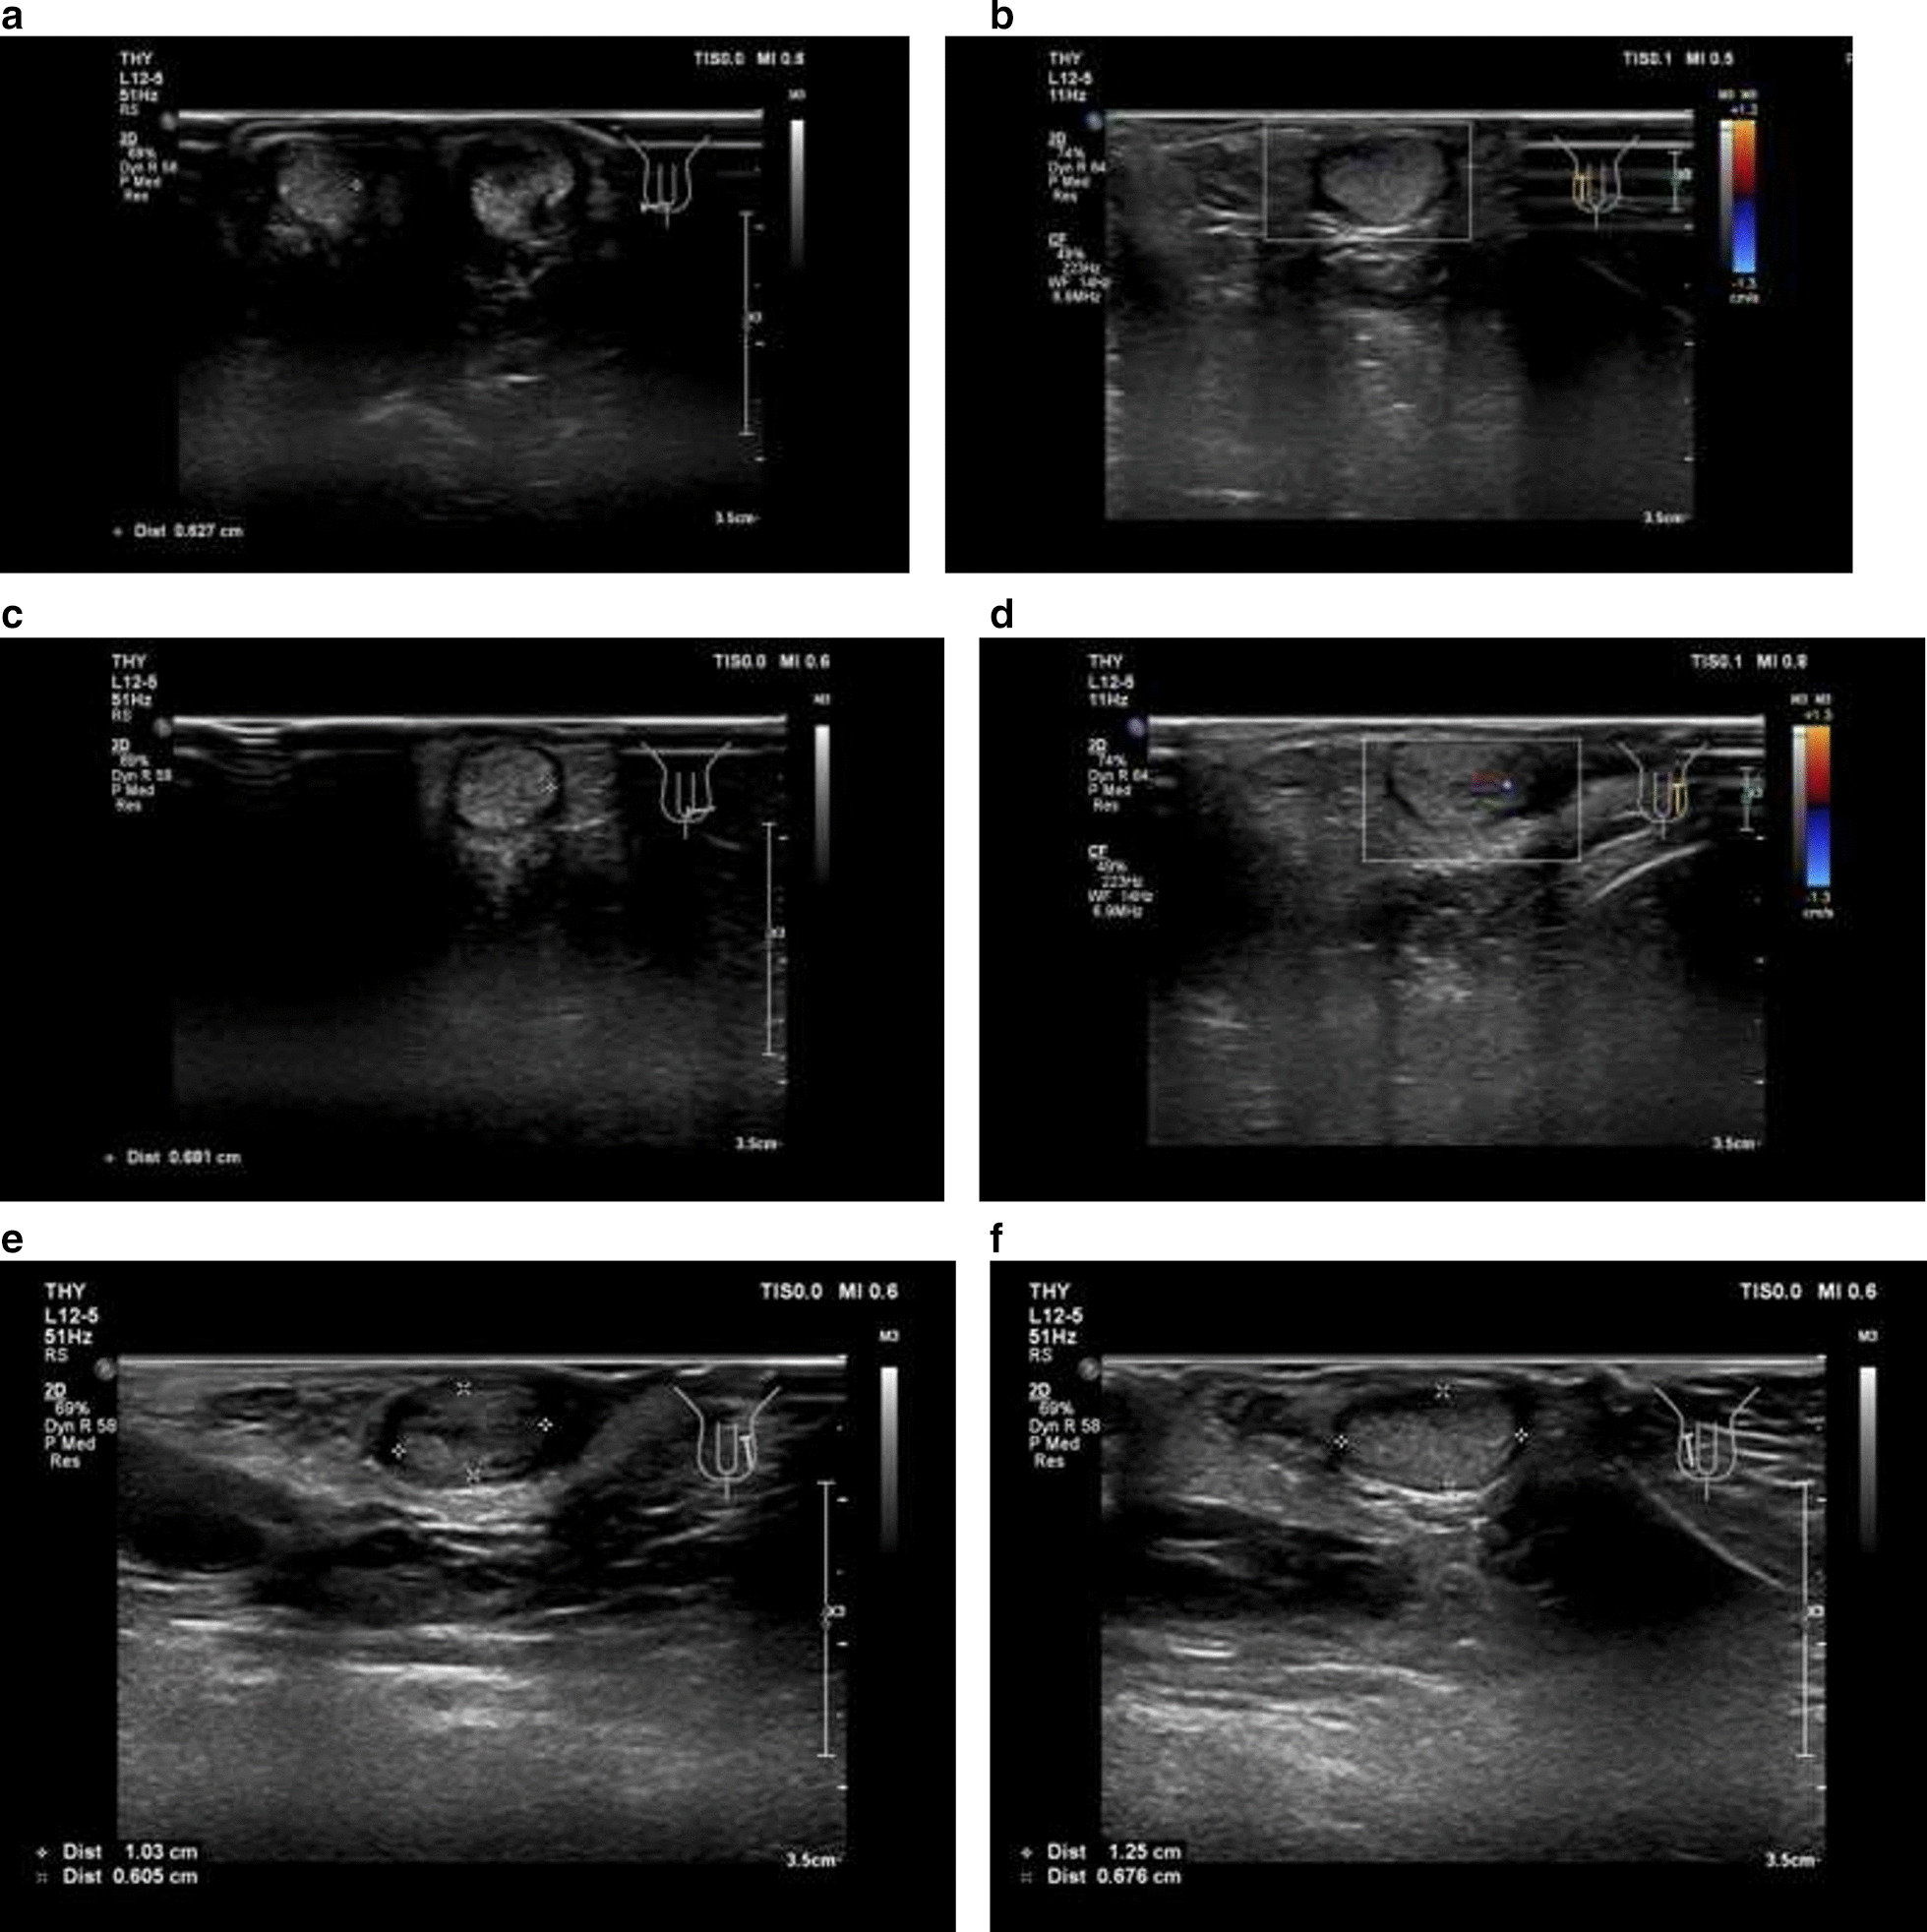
Post-operative ultrasound of the testes. a The right testis; b post-operative Doppler image of blood supply for the right testis; c left testis; d post-operative Doppler image of blood supply for the left testis; e and f follow-up ultrasound 1 year after the surgery showed that the left and right testes had a size of 1.0 × 0.6 × 0.6 cm and 1.3 × 0.7 × 0.7 cm, respectively, both with a homogeneous echogenic appearance and normal blood supply
